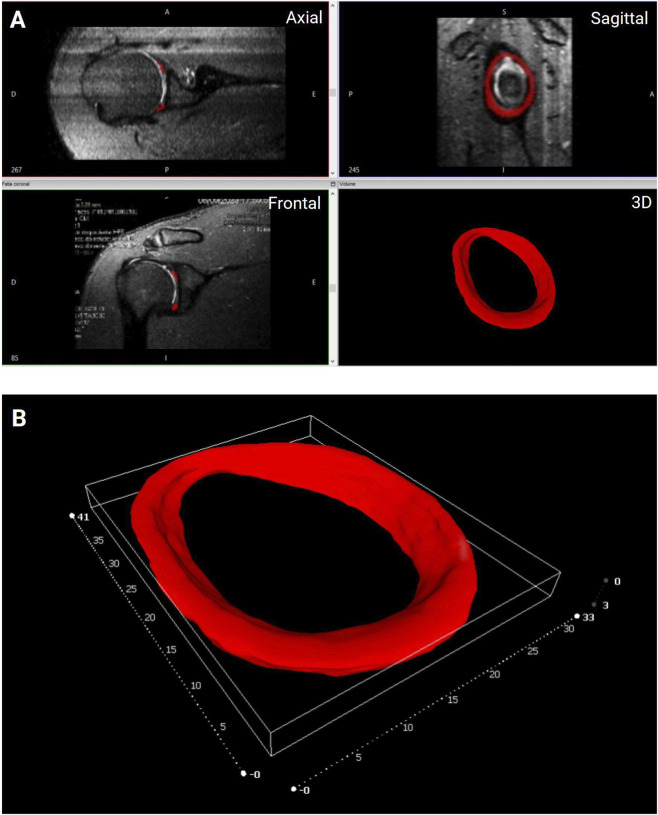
Generation of the 3D digital model of the glenoid labrum based on magnetic resonance imaging data. (A) Segmentation of the labrum in InVesalius software, showing the anatomical isolation in axial, sagittal, and frontal planes, and the corresponding 3D reconstruction. (B) Final volumetric rendering of the 3D model, representing the patient-specific geometry of the glenoid labrum used for subsequent printing steps. Scale in millimeters.
